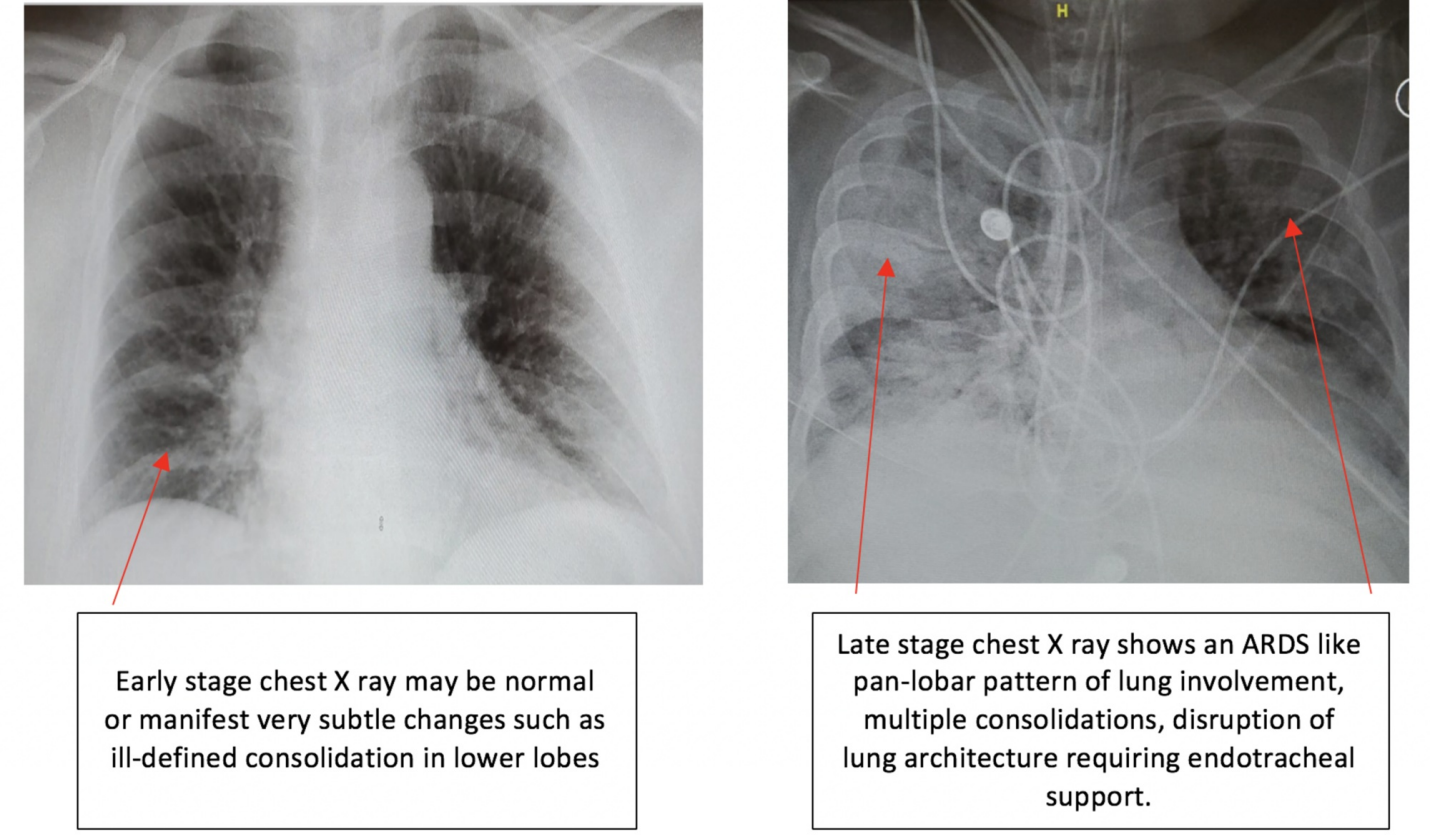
Early and late-stage X-ray findings in patients with COVID-19 ARDS - acute respiratory distress syndrome
